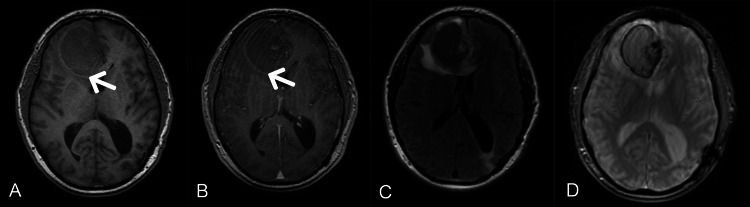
MRI brain: The T1-weighted axial image without contrast (A) shows peripheral T1 hyperintensity and heterogeneous mildly increased T1 signal internally. T1 post-contrast (B) reveals an ovoid right frontal lobe mass, predominantly isointense to the cortex, with peripheral T1 hyperintensity (white arrows) and mild central nodular heterogeneous enhancement. The T2 FLAIR (C) demonstrates the mass, which mostly exhibits low signal, with a nodule of increased signal. There is associated peri-lesional vasogenic edema and mass effect, with midline shift involving the bilateral frontal horns of the lateral ventricles. The gradient recalled echo (GRE) image (D) shows peripheral and internal foci of blooming, suggestive of hemorrhage.
